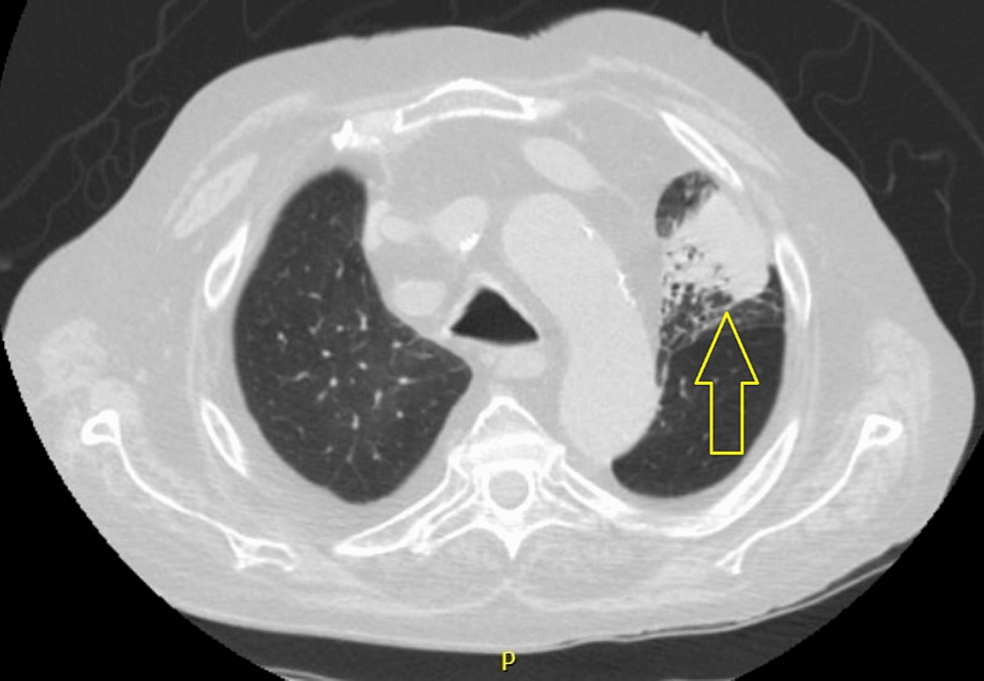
Chest CT-scan showing left upper lobe atelectasis
